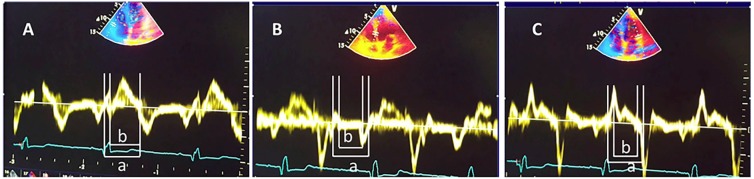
Tissue Doppler myocardial performance index measurement. Samples
